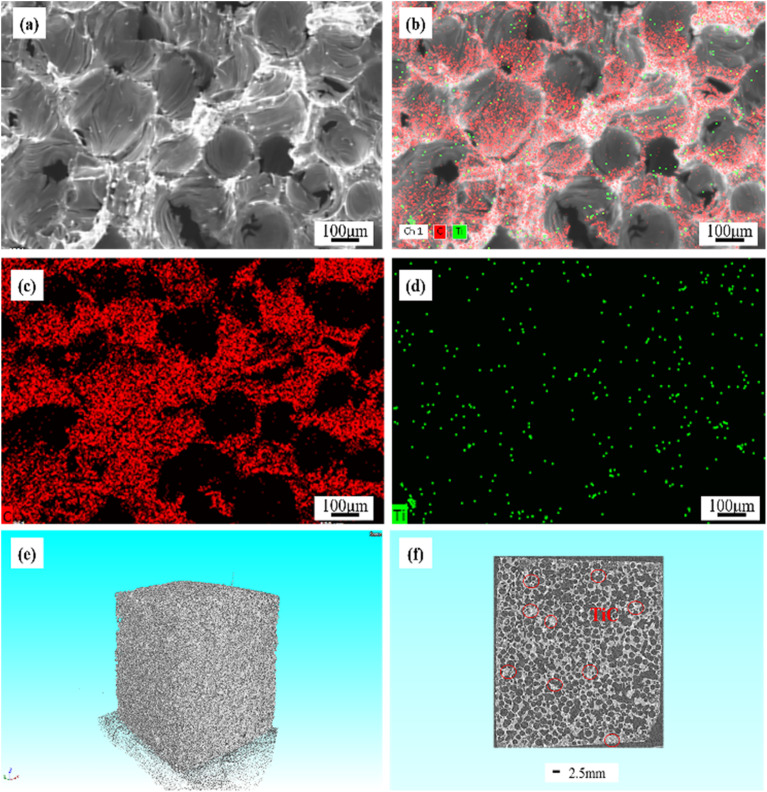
EDS mapping analysis (a)–(d) of GF-11, high-resolution CT images of GF-11 (e and f).
